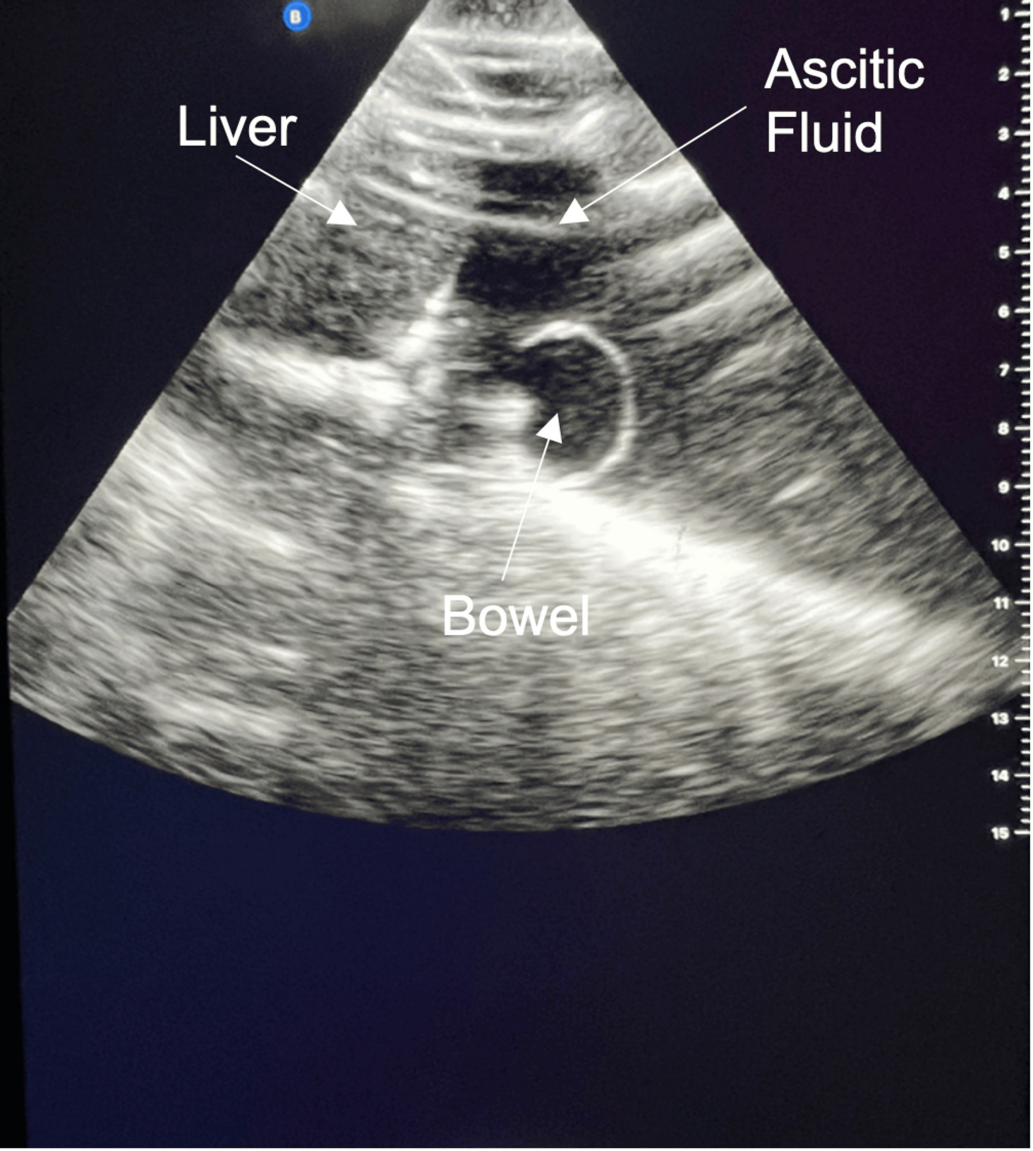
USGAP model under ultrasound showing presence of liver, bowel and ascitic fluid labelled Depth is shown on the right-hand side in centimetres USGAP: ultrasound-guided abdominal paracentesis
